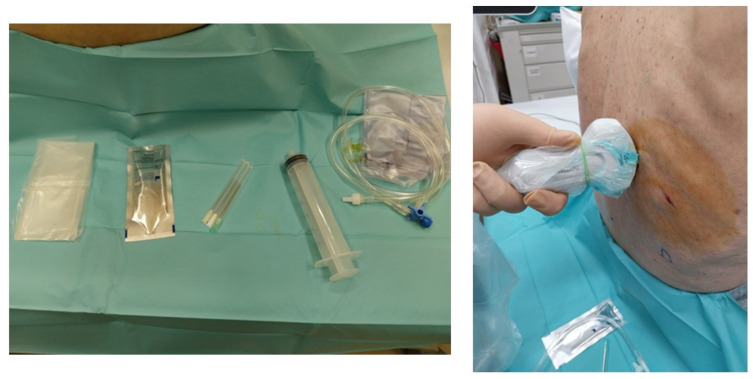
Example of a thoracentesis kit: the kit includes 3 needles of different caliber, a connection system with a 3-way tap, a syringe for aspiration, and a collection bag. On the left side, there is a sterile probe cover and a sterile gel pack. In the best operating setting with a mobilizable and cooperative patient, the patient sits on the edge of the bed and hugs a pillow; this movement allows the intercostal spaces to open more easily. A sterile field with a sterile drape is set up. The operator wears sterile gown and gloves. In this figure, the needle insertion site has already been identified through ultrasound evaluation, and local disinfection is carried out with povidone iodine by using sterile gauze.
